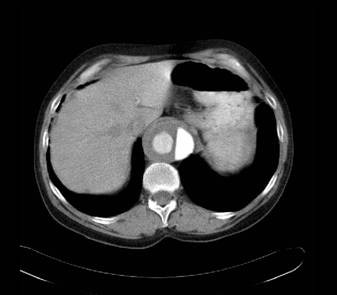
CT scans demonstrating the dissection at the level of the renal arteries.
